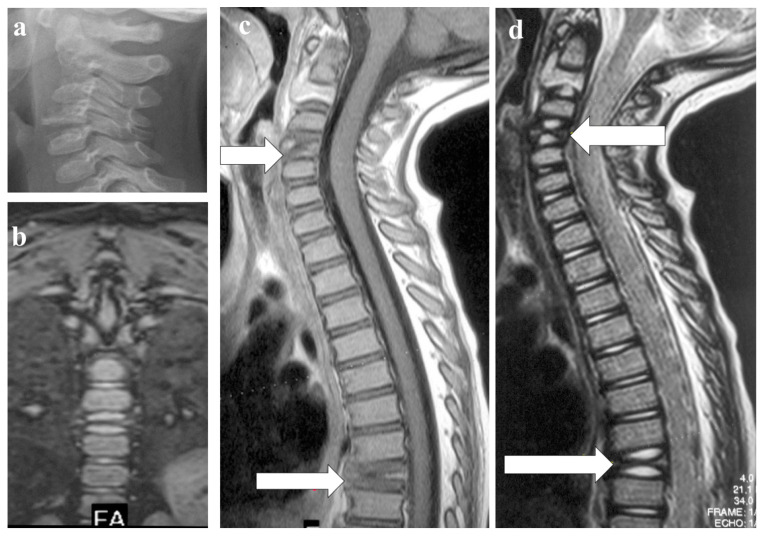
Langerhans cell histiocytosis in a 24-year-old male. (a) Cervical X-ray in lateral view shows vertebra plana in C4. (b) MRI of the cervical and thoracic spine demonstrates further vertebra plana in T8. Both vertebrae present flattening of the anterior body (with arrows) without compression of the spinal cord. (c) T1 sequence. (d) T2 sequence.
